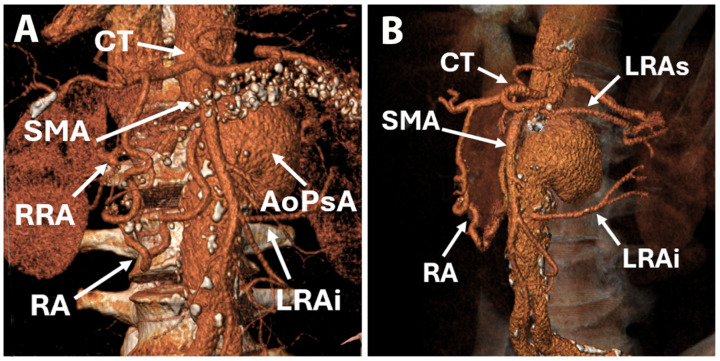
Computer tomography reconstruction of abdominal aorta and branches: anterior view (A), side view (B). AoPsA—aortic pseudoaneurysm; CT—celiak trunck; LRAi—left renal artery inferior branch; LRAs—left renal artery superior branch; RA—Riolan arcade; RRA—right renal artery; SMA—superior mesenteric artery.
